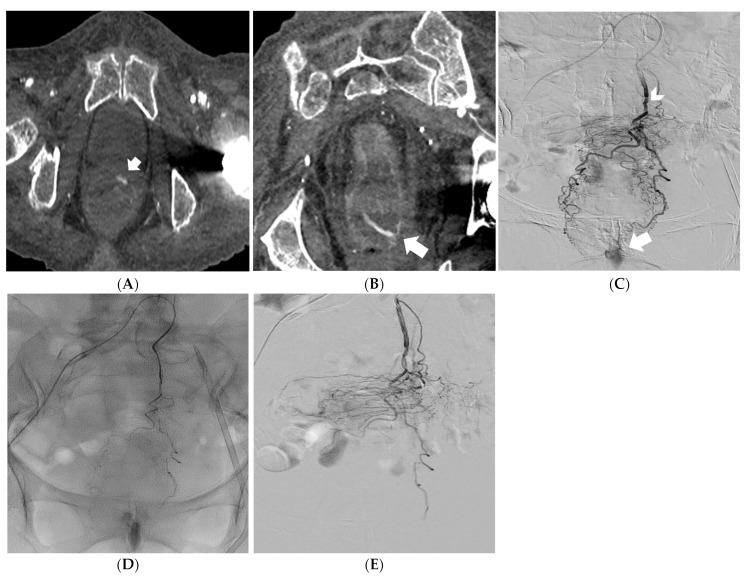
Reformatted CT angiography images in the oblique axial and coronal planes showing a massive rectal hemorrhage (arrows) (A,B). Digital subtraction angiography identifying the superior rectal artery as the feeding vessel (arrowhead); active bleeding is also noted (arrow) (C). Embolization of the superior rectal artery with PVA particles mixed with iodinated contrast media under fluoroscopy control (D). Digital subtraction angiography confirming successful embolization of the feeding vessel (patency of the middle and inferior rectal arteries, fed by the internal iliac arteries, prevented significant ischemic injury of the rectal wall) (E).
